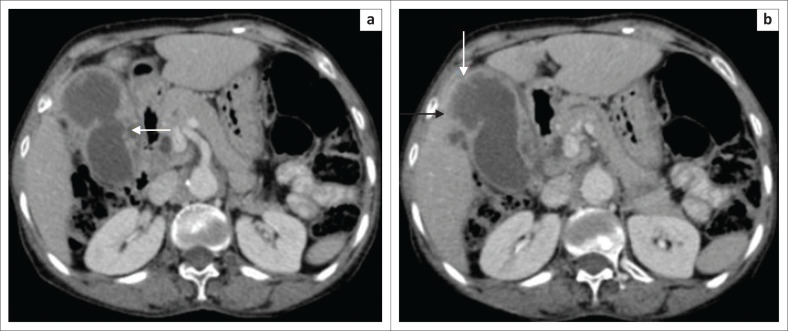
(a) Nodules appear hypodense on contrast-enhanced computed tomography images (arrow). (b) Focal breach in mucosal lining (white arrow) and indistinctiveness with adjacent liver noted (black arrow).
